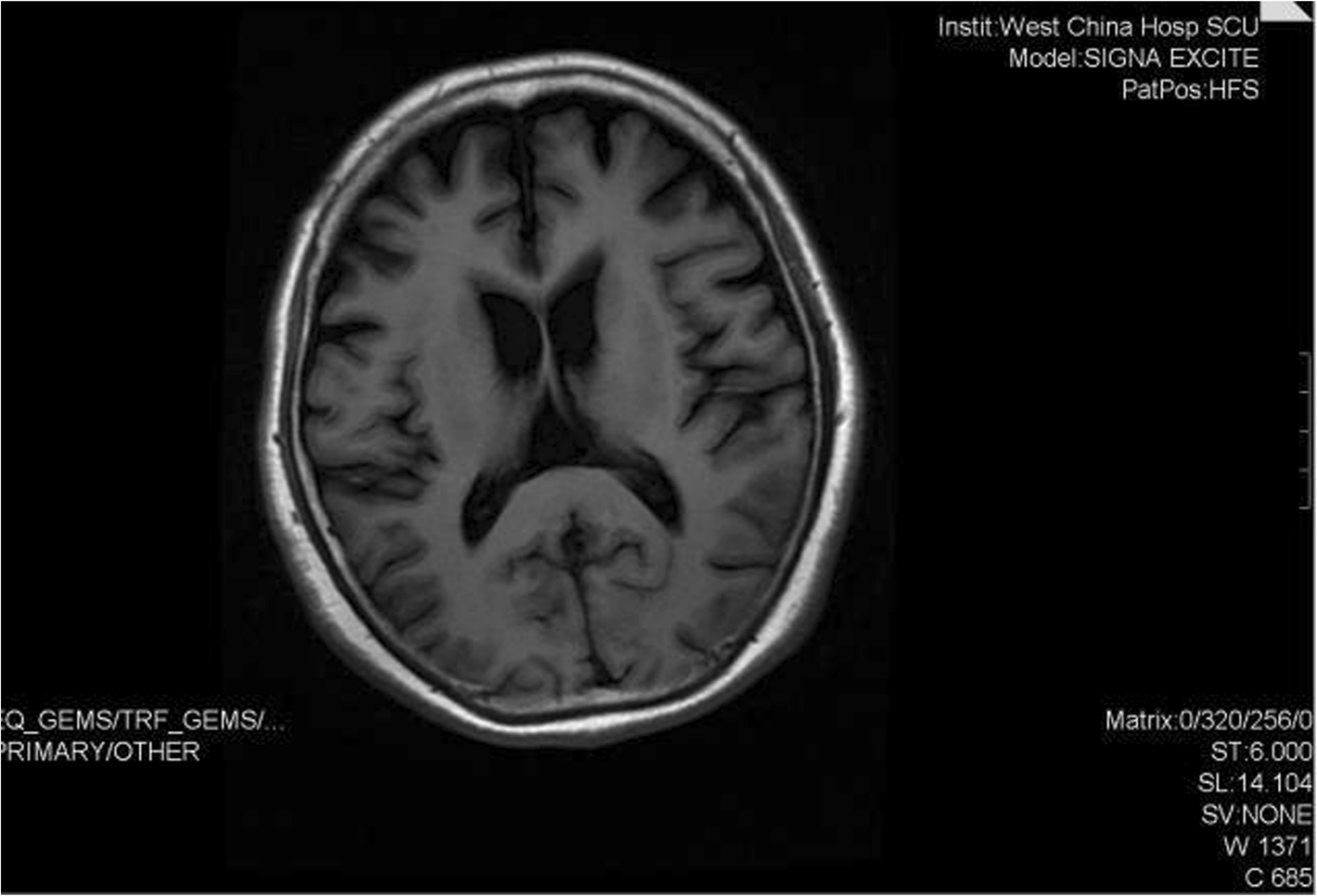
The brain MRI in September 2018. Brain MRI showed mild bilateral frontotemporal atrophy
